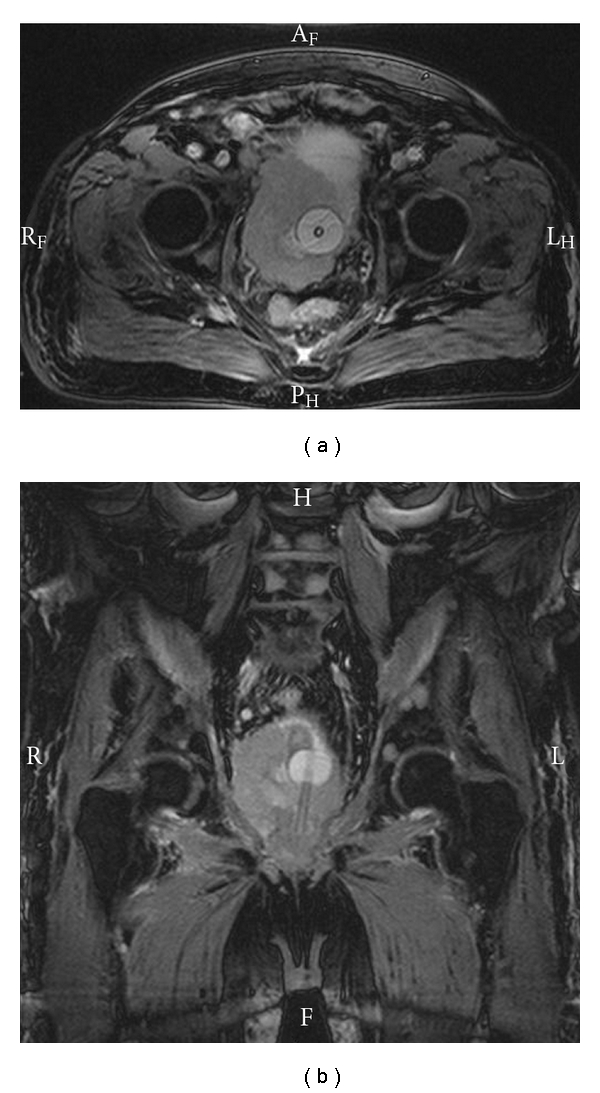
MRI demonstrating a local solid tumour with a diameter of 9 cm infiltrating the trigone and the bladder floor on each side.
